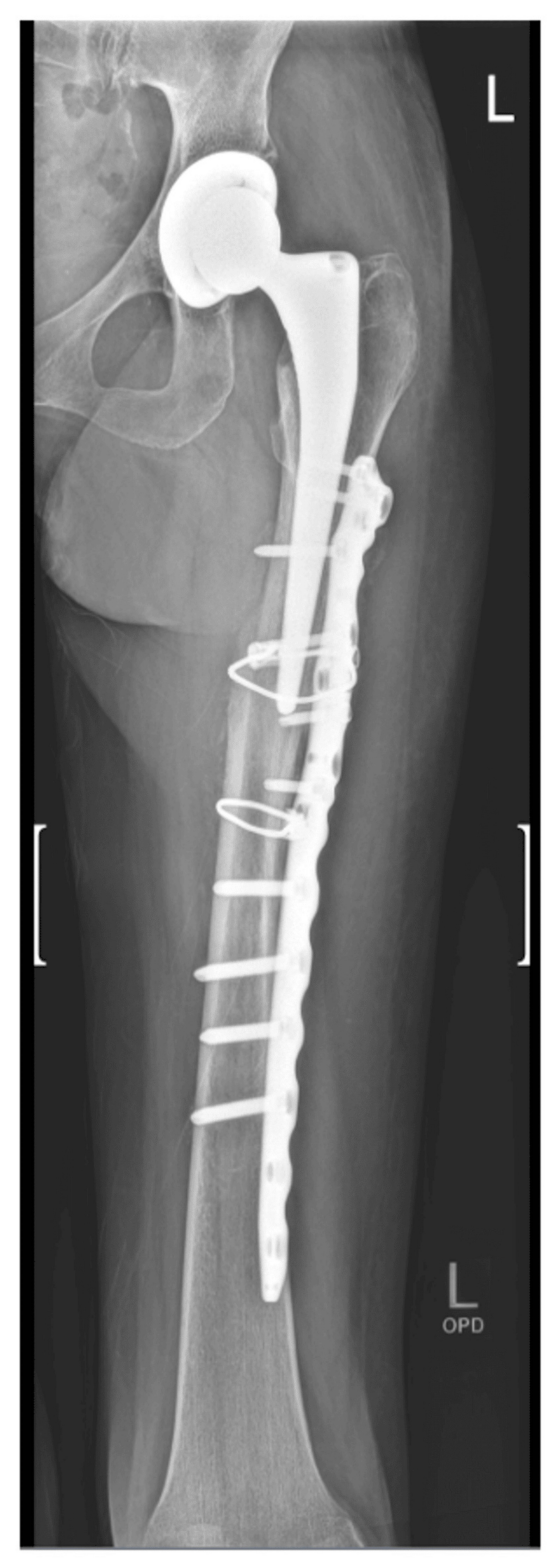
Left femoral anterior-posterior X-ray showing a periprosthetic fracture of the stem in a 54-year-old female patient
